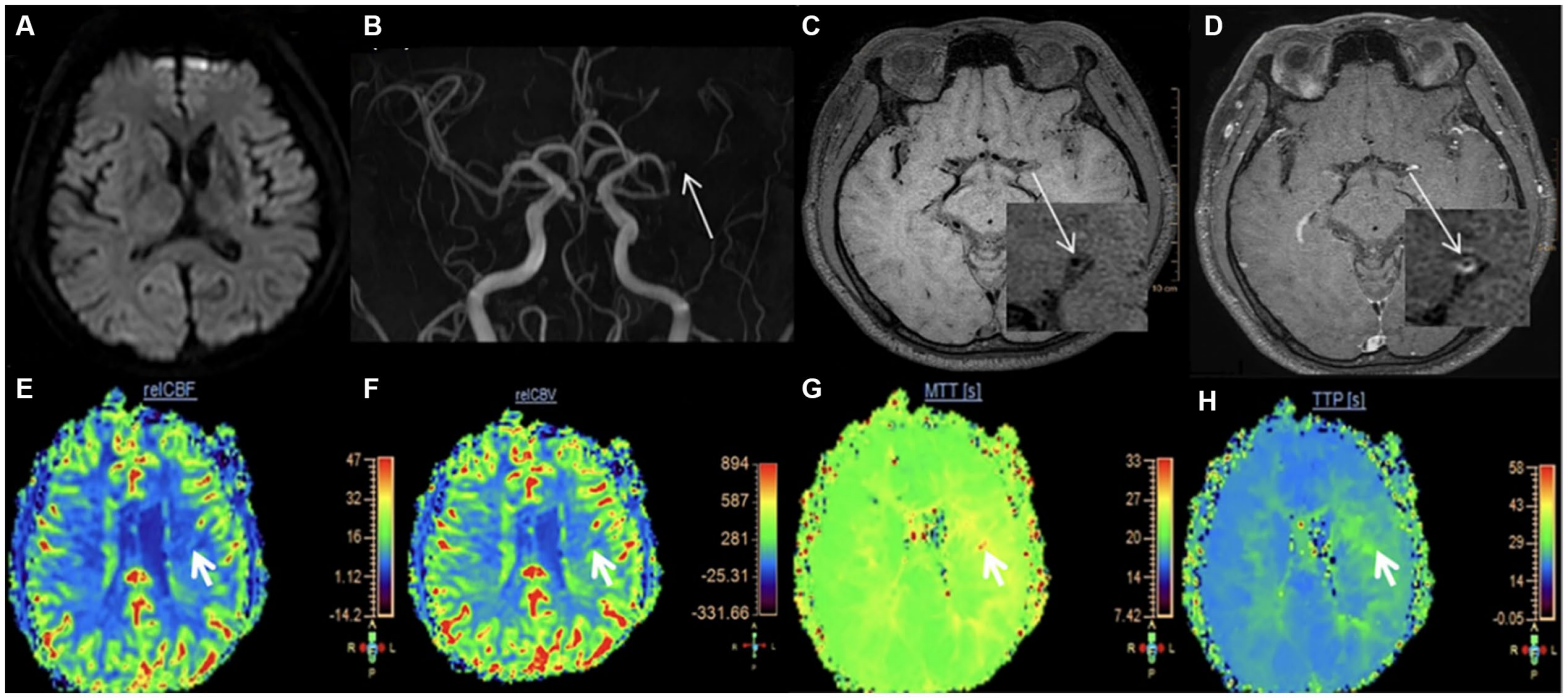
Measurement of vessel and plaque characteristics in hypoperfusion. A 32-year-old male was admitted to our hospital for 4 h due to right limb weakness. (A) DWI is normal; (B) The stenotic site of the left MCA. The white arrow shows the stenotic site of the left MCA; (C) T1-weighted HR-VWI imaging shows the plaque in the left MCA. The white arrow shows the short-axis view; (D) Enhanced T1-weighted HR-VWI imaging showing the measurement at the most narrowed site in left MCA. OWA = 2.04 mm^2^, IWA = 0.89 mm^2^. (E–H) The four pseudo-color maps of rCBF, rCBV, MTT, and TTP are shown respectively, with the white arrow indicating areas of hypoperfusion. rCBF = 5.86, rCBV = 156.84, MTT = 26.78 s, TTP = 26.84 s, rrCBF = 71.19%. DWI, diffusion-weighted imaging; MCA, middle cerebral artery; HR-VWI, high-resolution vessel wall imaging; OWA, outer wall area; IWA, inter wall area.
